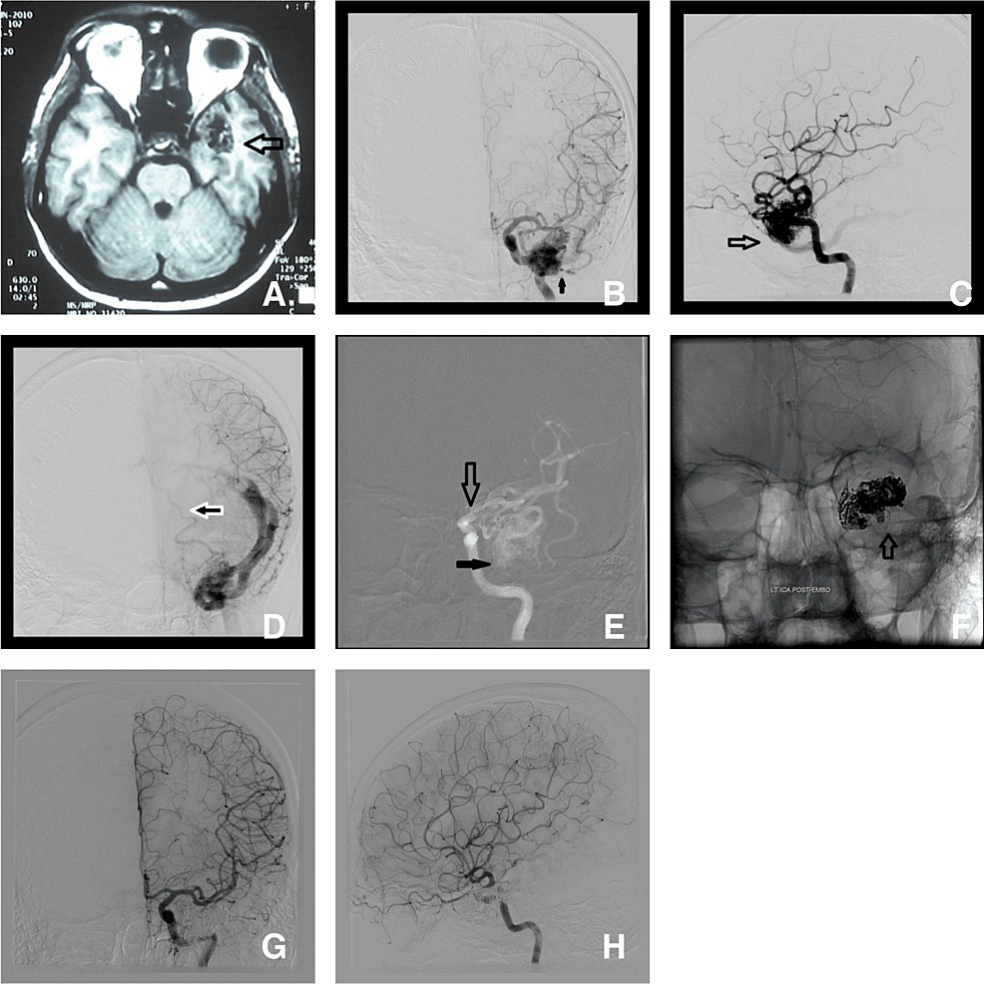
MRI axial plane (A) shows the left anterior temporal AVM. DSA images anteroposterior view (B) and lateral view (C) show the AVM nidus (empty black arrow). Image (D) anteroposterior view shows the deep Sylvian vein draining into the basal vein of Rosenthal (white-bordered black arrow). Image (E) shows the microcatheter taking multiple acute curves (empty black arrow) and the tip of the microcatheter just at the entrance of the nidus (solid black arrow). Image (F) anteroposterior view shows the Onyx cast. DSA anteroposterior view (G) and lateral view (H) show complete obliteration of the AVM in the immediate post-embolization stage. DSA after three years confirmed a complete cure (not shown here). AVM - arteriovenous malformation; DSA - digital subtraction angiogram
